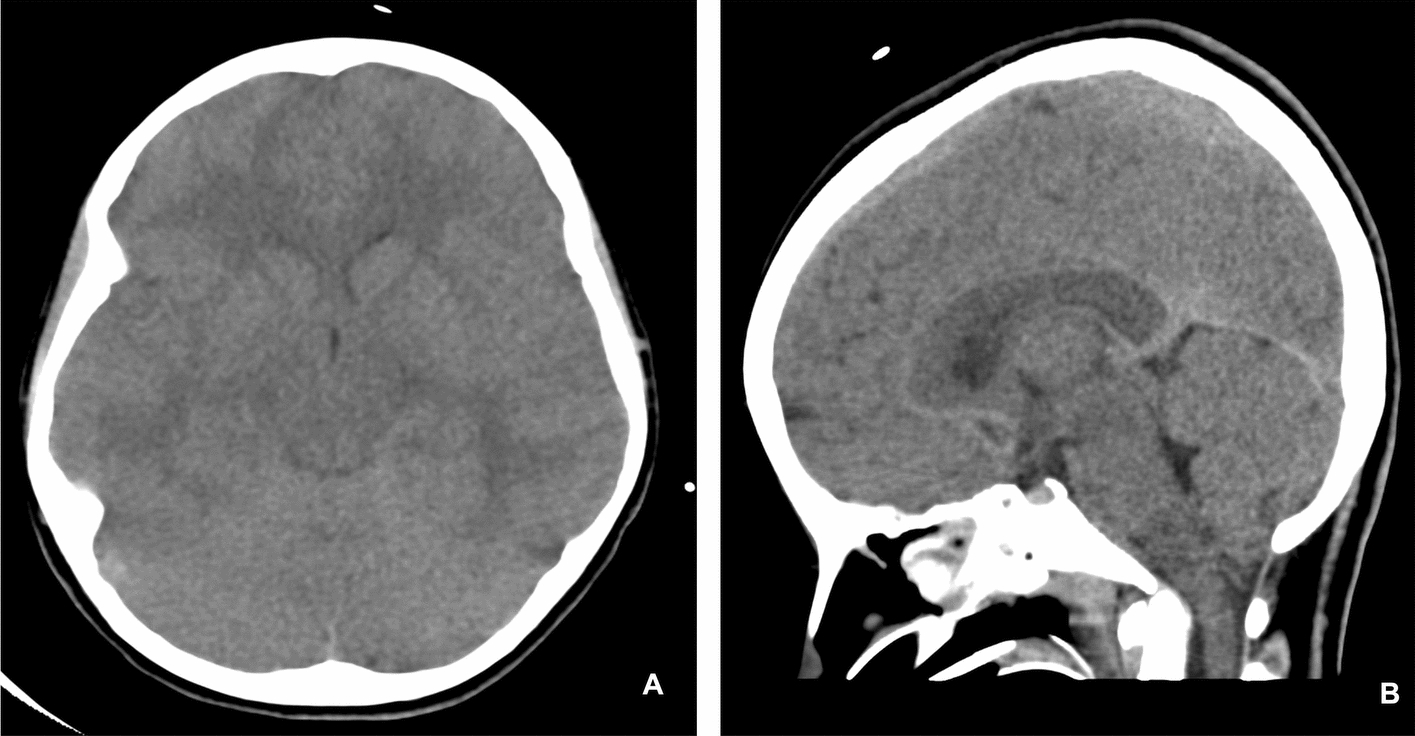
Unenhanced computed tomography scan follow-up in 24 hours A Axial image showing interval improvement of the brain edema. B Sagittal image showing interval improvement of the tonsillar herniation
